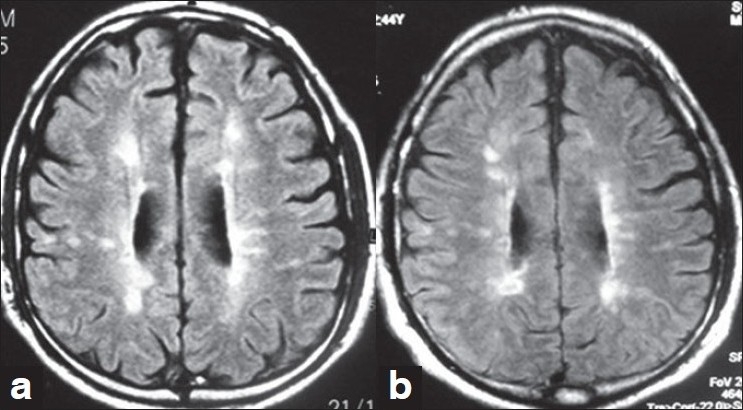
(a) Baseline MRI brain of patient with SPMS before starting IFN β. (b) Follow up MRI brain of patient with SPMS after starting IFN β
